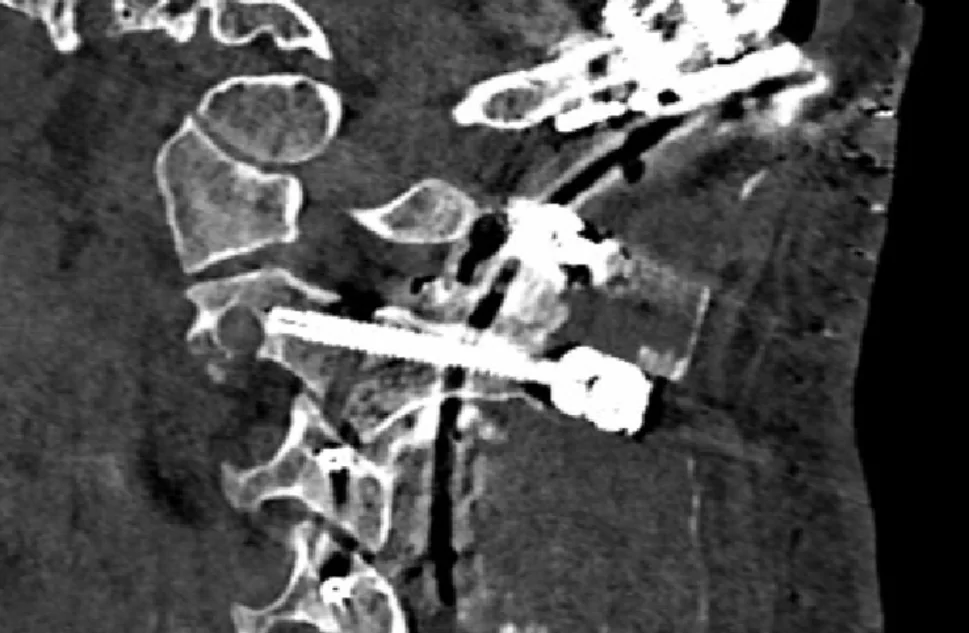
Sagittal CT pedicle screw C2-rt. (same patient as in figures 3–5 and 7–9). *C*,* cervical spine*
